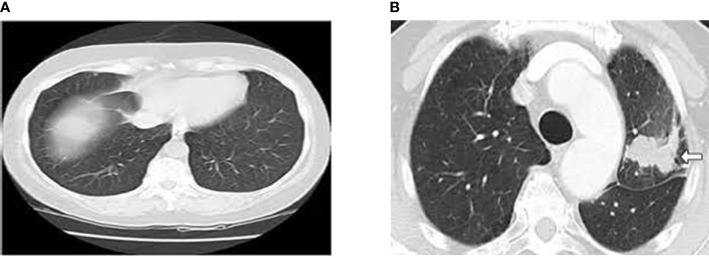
(A) Normal CT Lung Image (B) Abnormal CT Lung Image (Cancer Image).
